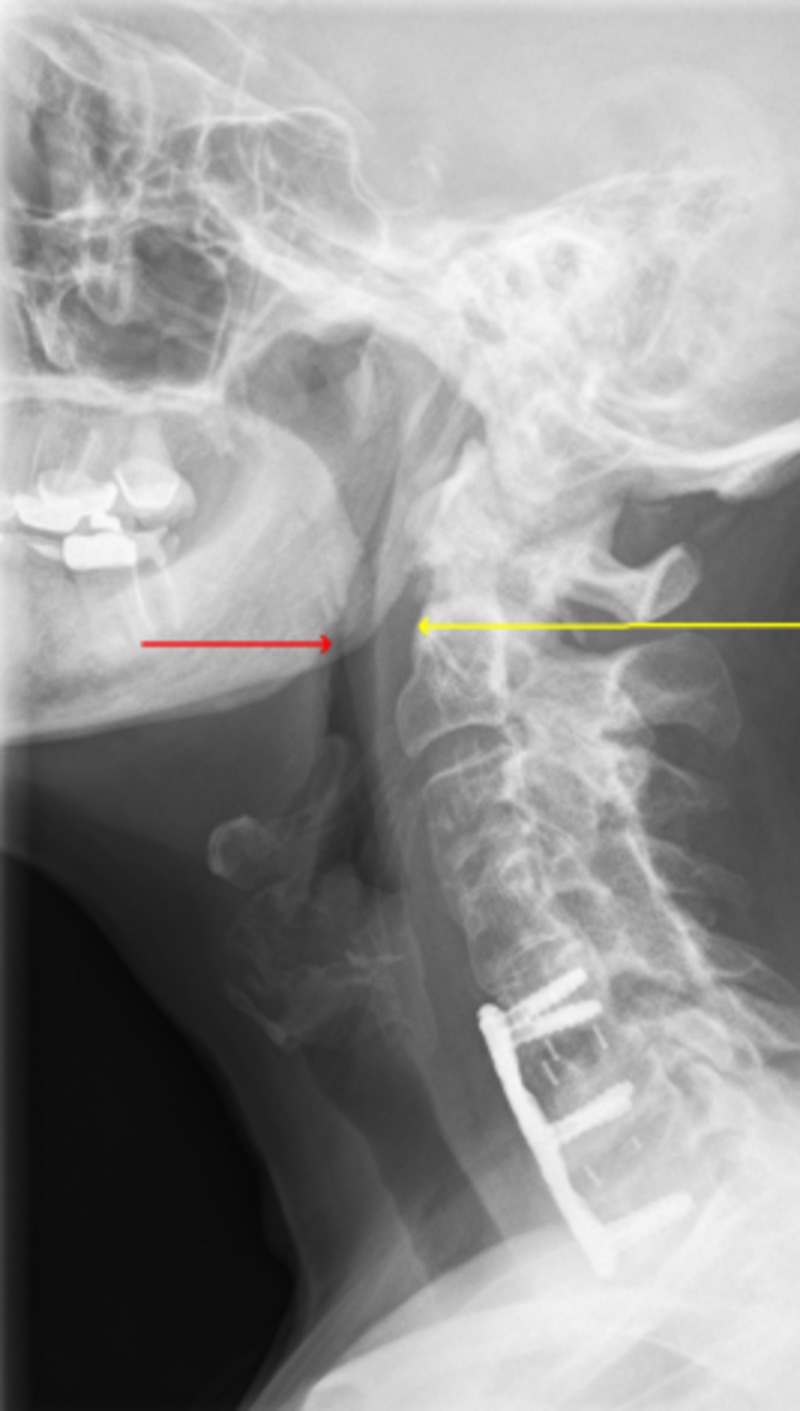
Postoperative cervical x-ray showing anterior plate and screw fixation at C5-7 with a narrowing airway (red and yellow arrows)
